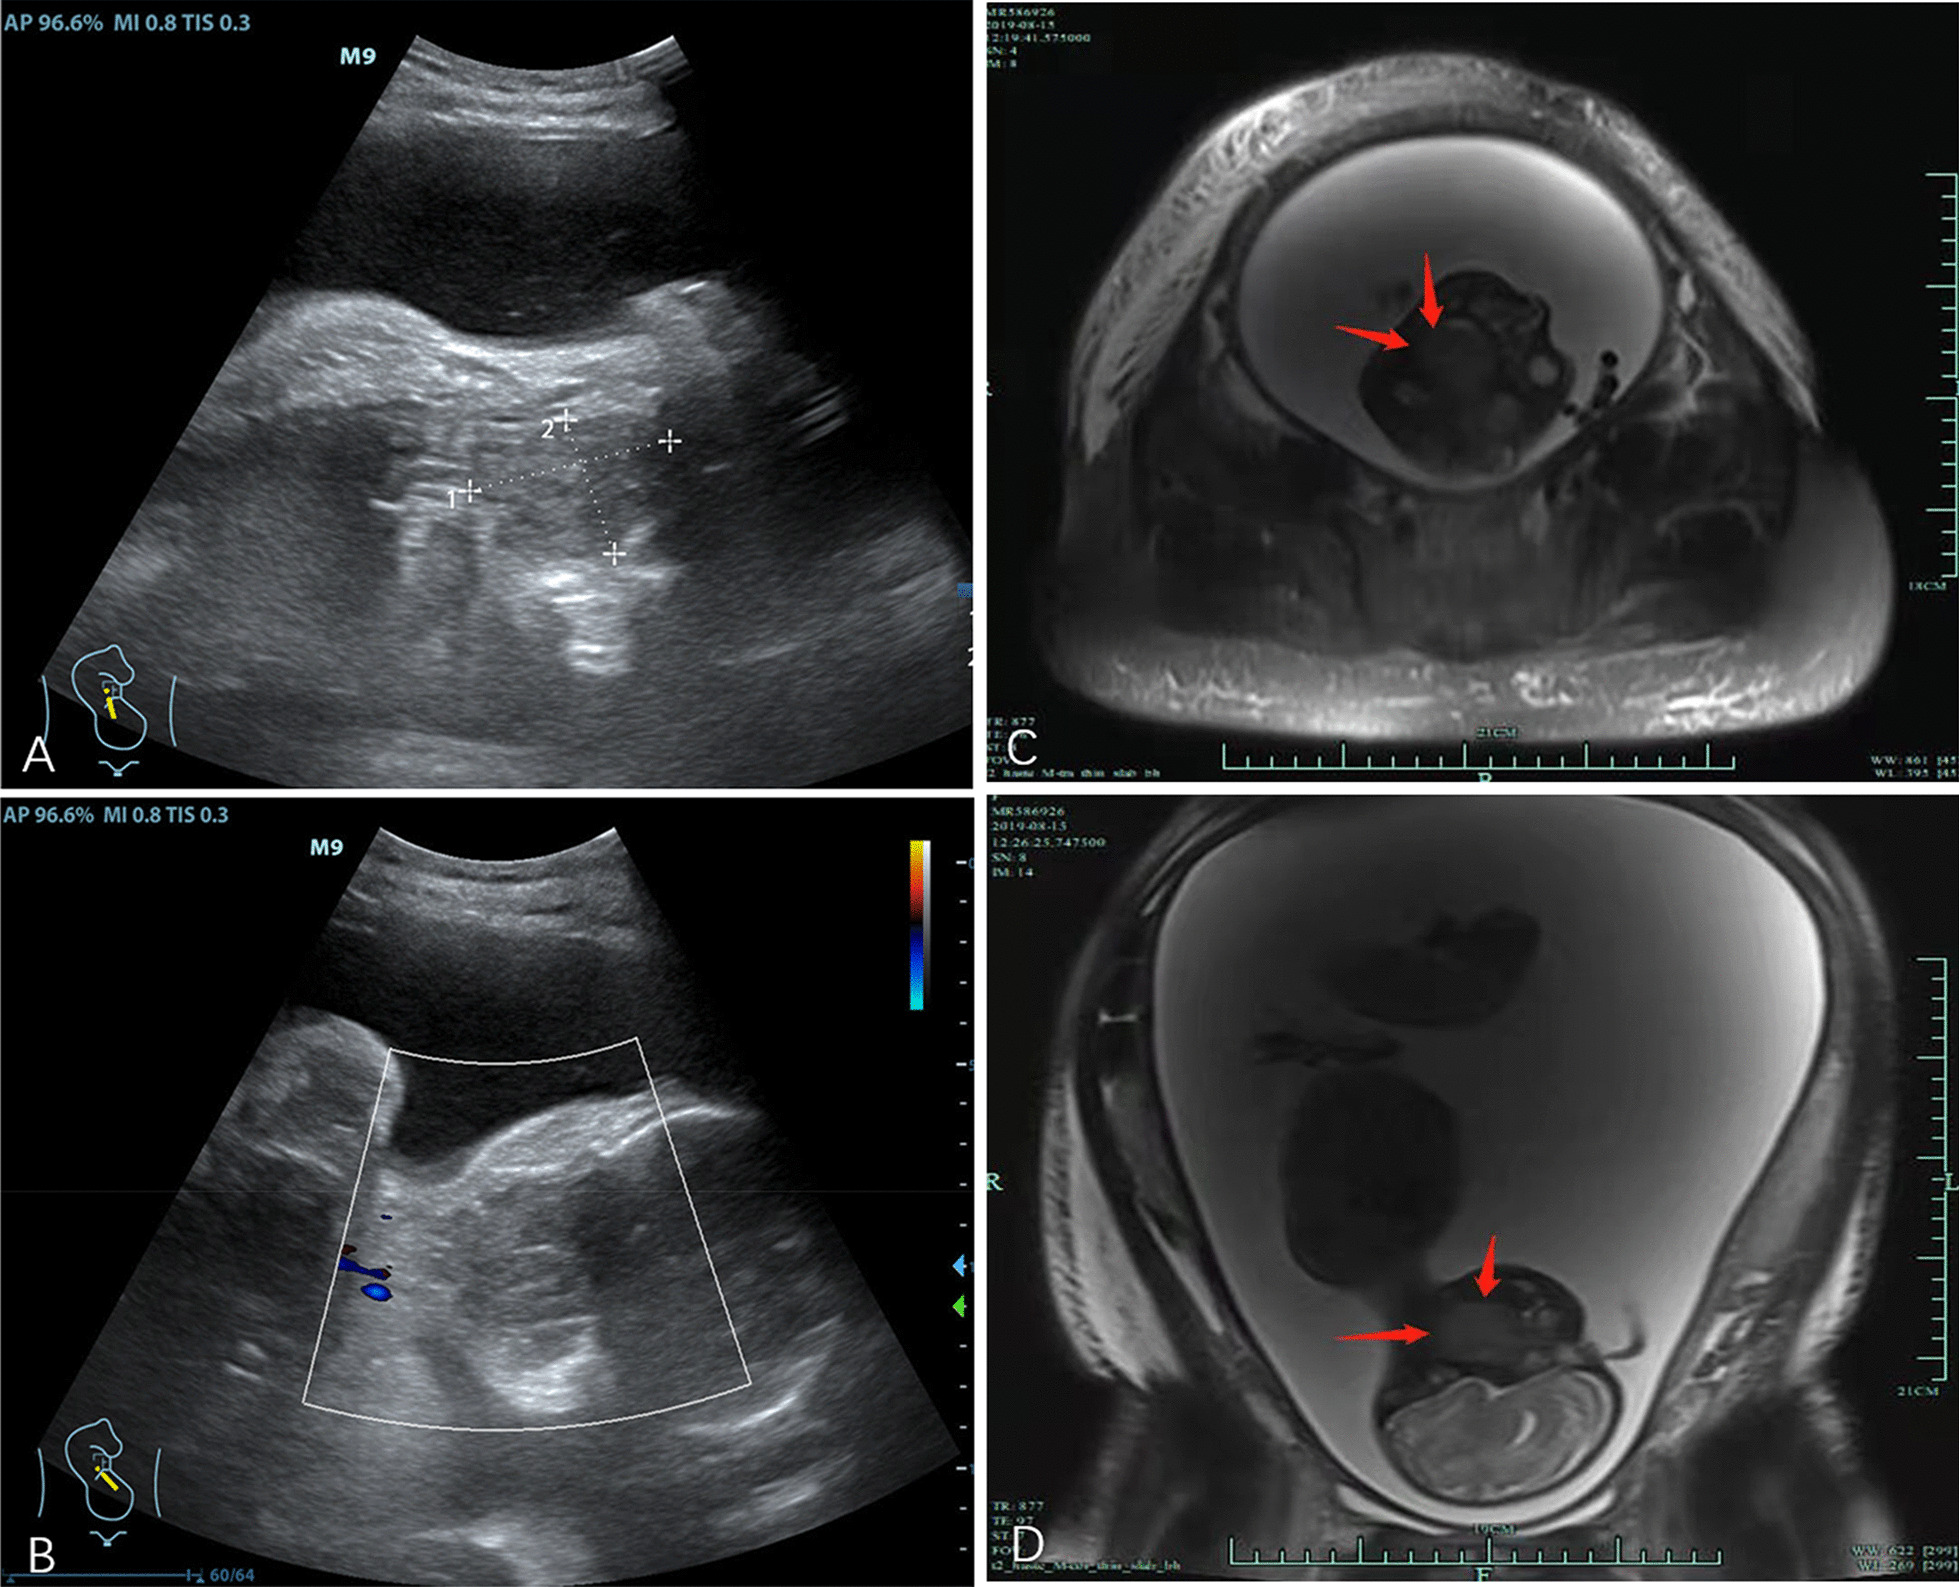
Ultrasound and MRI images of pharyngeal glial heterotopia. A, B Sonographic examination demonstrating a slightly hypoechoic mass (5.4 cm × 4.4 cm × 3.4 cm) in the pharynx of the fetus with a clear boundary and without detectable blood flow. C, D Pelvic magnetic resonance imaging (MRI) revealed a solid mass (4.5 cm × 3.7 cm × 3.3 cm) with a complete capsule and slight hyperintensity on T2WI in the fetal pharynx
